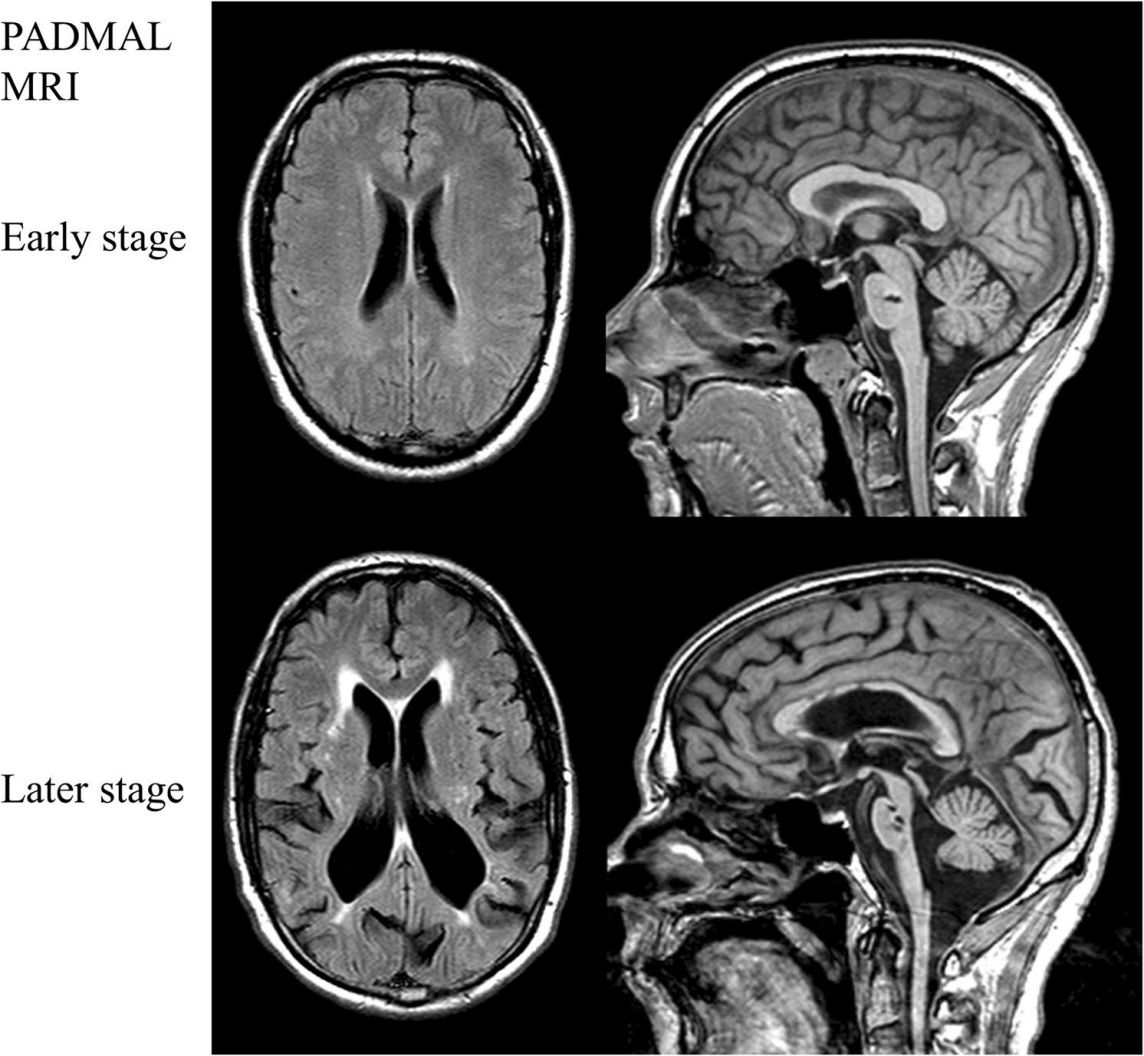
MRI features of PADMAL. T2-weighted cranial MRI scans of an early and a later stage PADMAL patient demonstrating pontine and supratentorial involvement
